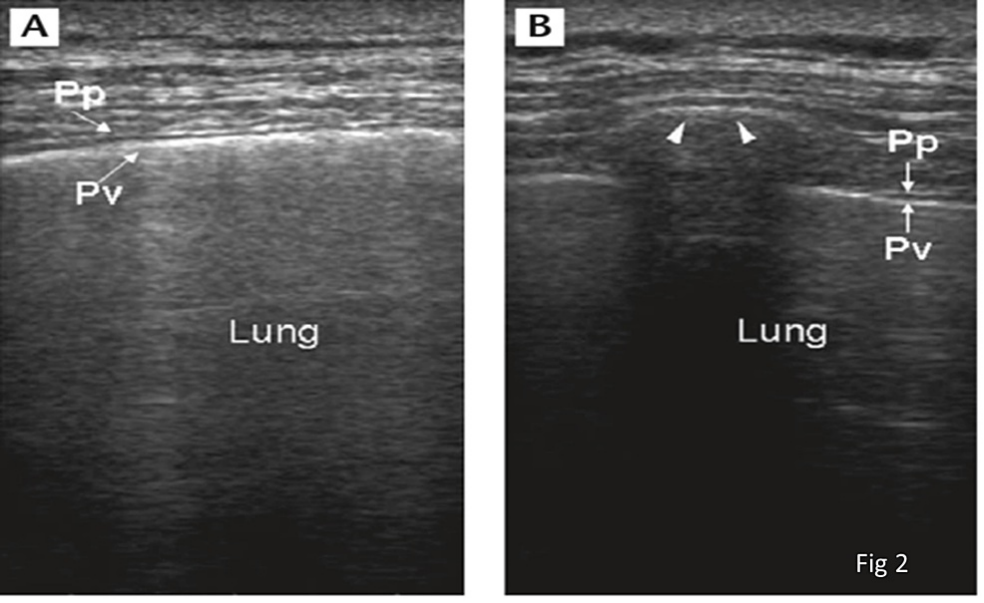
Normal chest ultrasound images (a) transverse scan of intercostal space; (b) longitudinal scan along the ribs. Pp= parietal pleura; Pv=visceral pleura. Image source: Reference no. [13]
